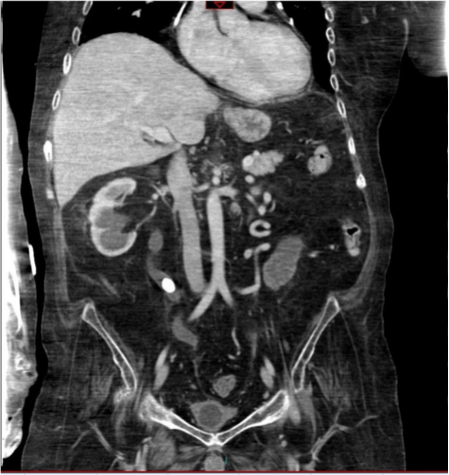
Follow-up CT, performed three months after cystoscopic clot evacuation: Coronal section shows complete resolution of bladder haematoma and bladder perforation.
